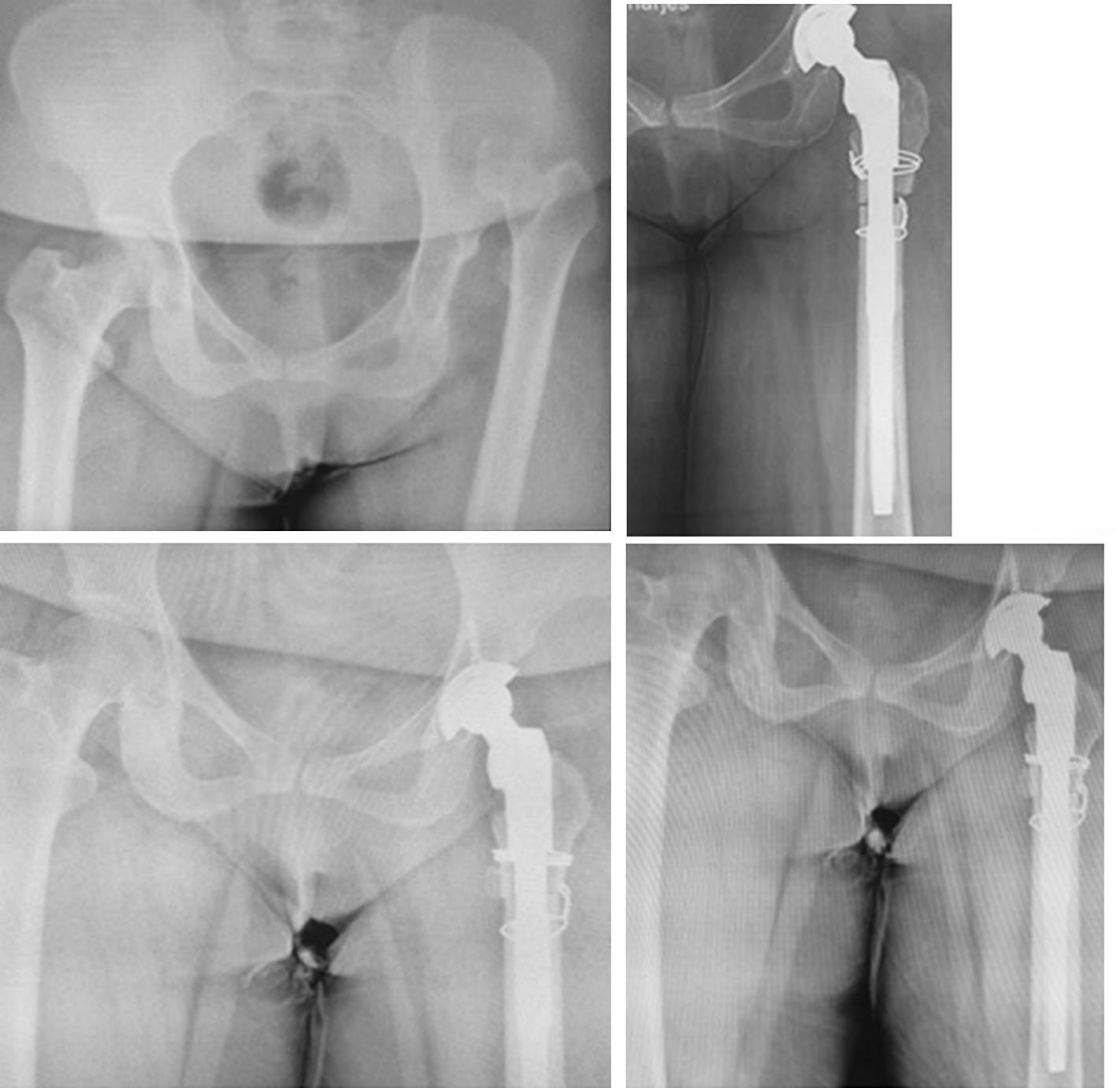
High-riding DDH undergone THR with cup placement in true acetabulum and transverse subtrochanteric osteotomy. Follow-up radiographs at 12th and 18th months reveal union of osteotomy site
